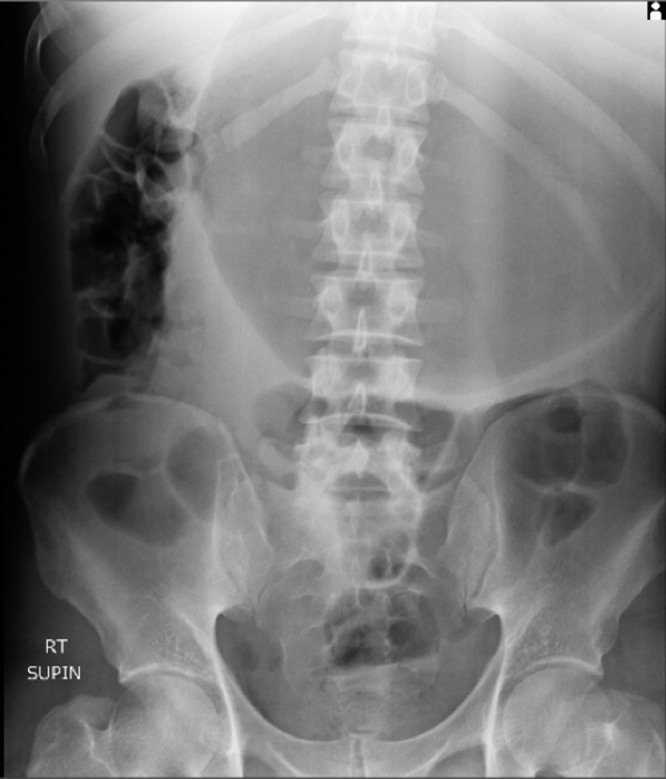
Abdominal X-ray (supine) shows a massively dilated stomach in an expected intraabdominal location with collapsed small bowel loops.
